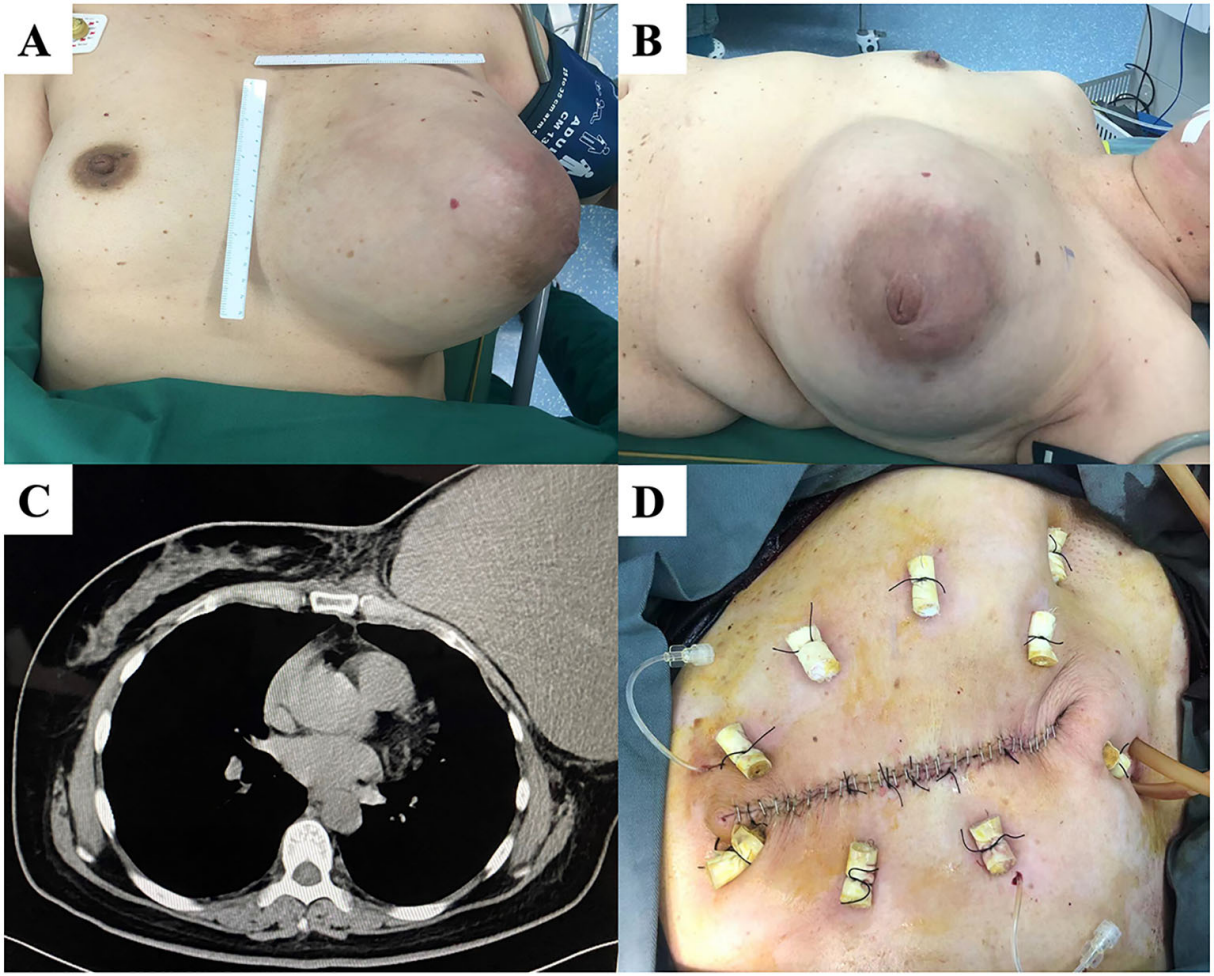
(A, B) A patient with a large left breast mass and intact and unbroken skin. (C) Chest computed tomography (CT) showing a clear demarcation of the mass from the chest wall. (D) Skin sutured after total mastectomy.
